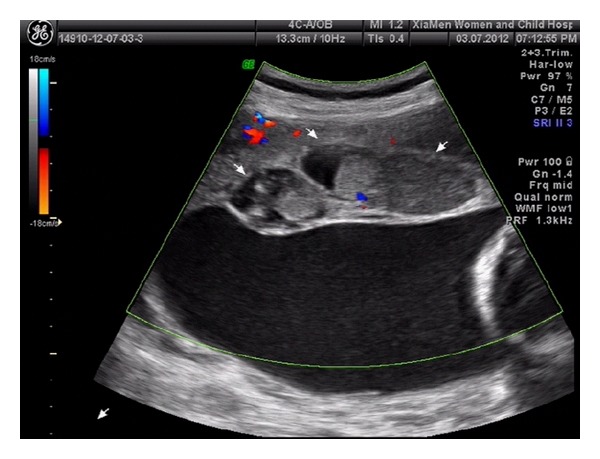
Transabdominal ultrasound showing a bulky placenta contained a mixed-echogenic mass measuring approximately 83 × 30 × 56 mm.
